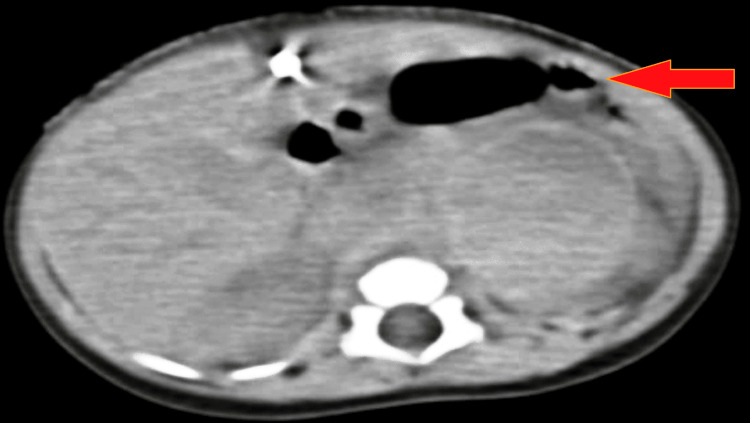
CT Scan of adrenal gland reveals left supra renal haemorrhage as shown by arrow
